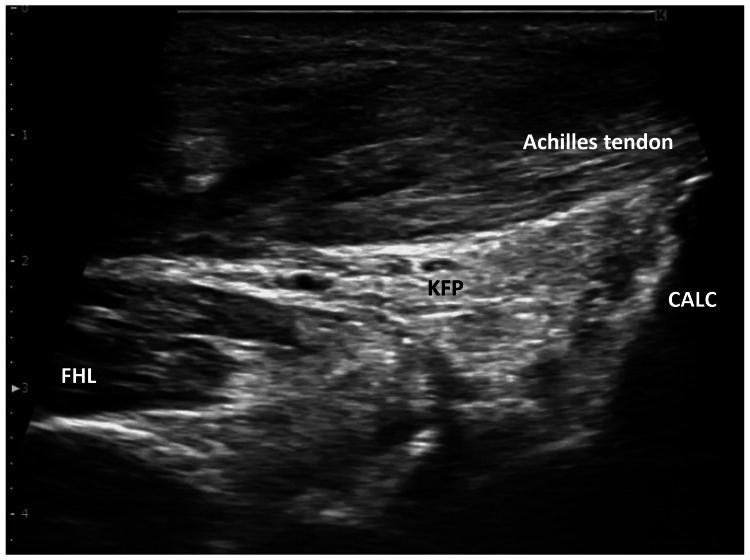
Static ultrasonography of the Achilles tendon after ultrasound-guided manual therapy (16-week post-surgery) CALC: calcaneus; FHL: flexor hallucis longus muscle; KFP: Kager’s fat pad.
